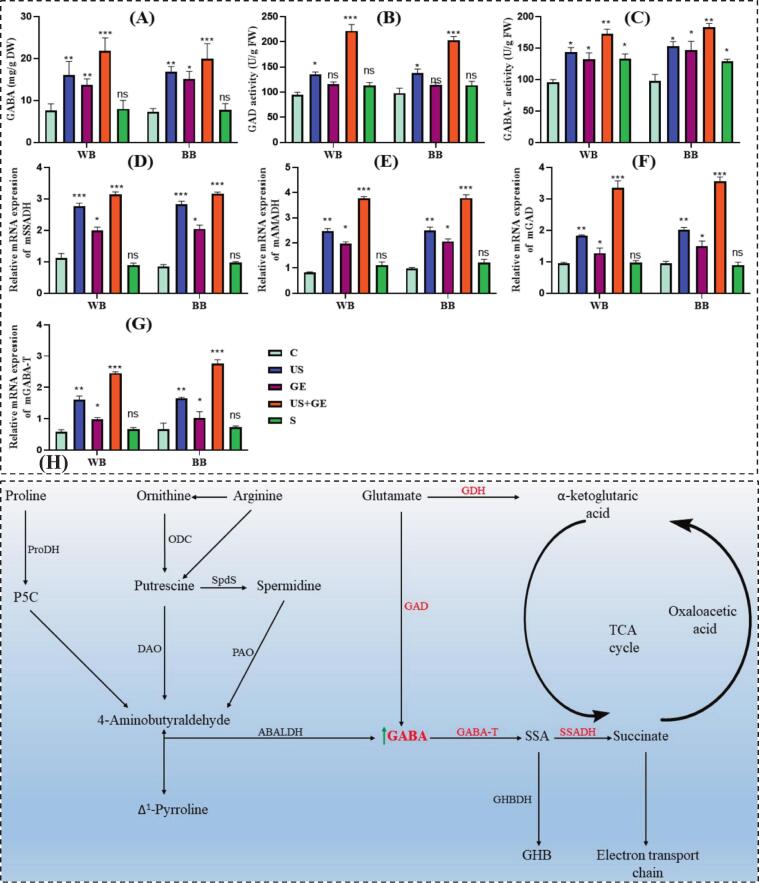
GABA accumulation and its relative gene expression in highland’s barley fractions subjected to ultrasonication, germination, ultrasound assisted germination and soaking. A. GABA contents of treated barley fractions. B. GAD activity of treated barley fractions. C. GABA-T activity of treated barley fractions. D. mRNA expression of mSSADH of treated barley fractions. E. mRNA expression of mAMADH of treated barley fractions. F. mRNA expression of mGAD of treated barley fractions. G. mRNA expression of mGABA-T of treated barley fractions. H. Metabolic pathway of GABA synthesis. C: untreated Tartary buckwheat, US: multifrequency ultrasonication, GE: germination, S: soaking. WB. Whole barley flour. BB. Barley bran. (*p* < 0.01; LSD), (n = 3).
